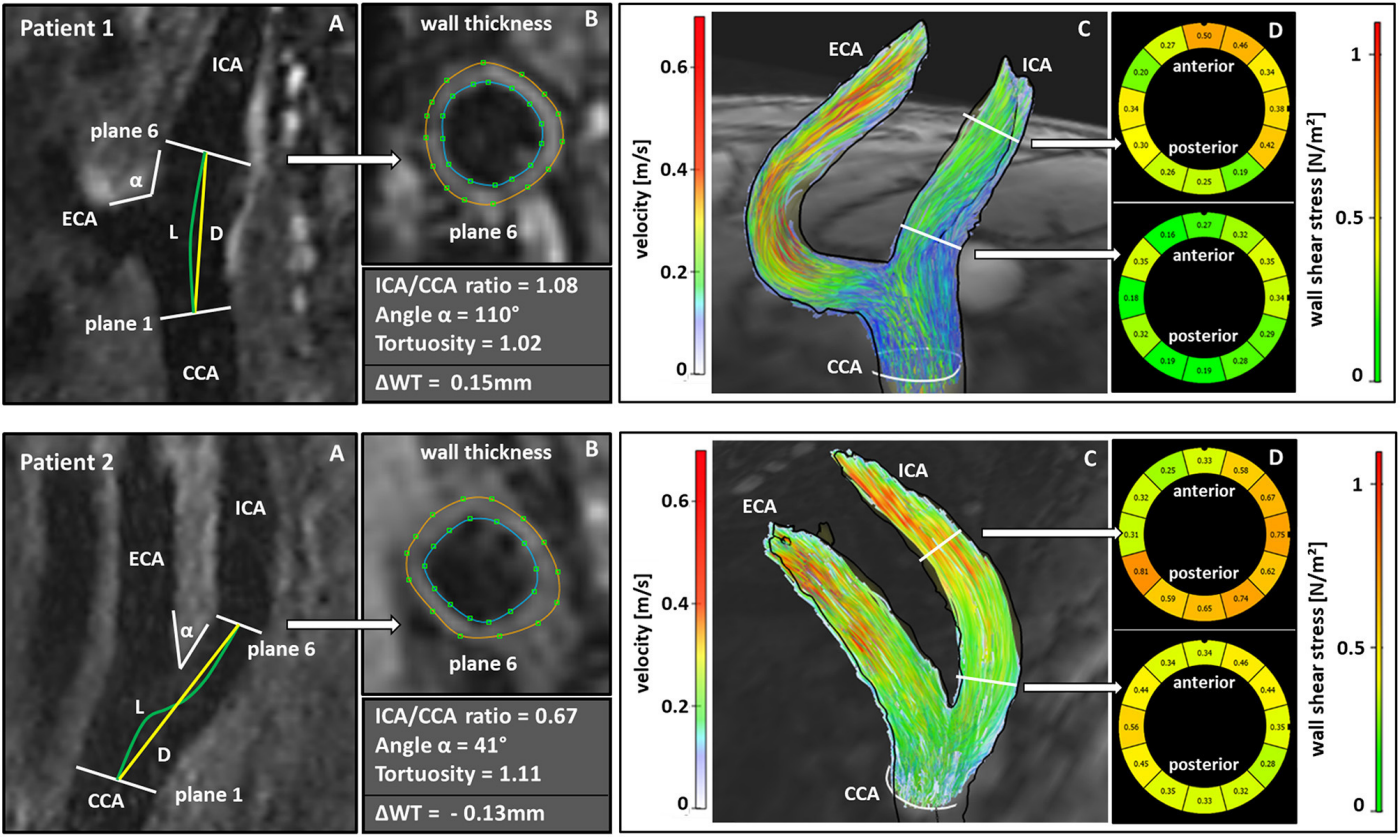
Comparison of geometric and hemodynamic properties associated with wall thickness progression (Patient 1) or plaque regression (Patient 2) during follow-up. Calculation of the ICA/CCA-ratio from the maximum diameters in plane 6 and plane1; L and D indicate the CCA-ICA distances along the lumen center (L) and the direct connection (D) for calculation of carotid tortuosity, α indicates bifurcation angle (A). Manual segmentation of wall thickness in the cross section corresponding to the white arrow (B). Streamlines represent absolute blood flow velocities in m/s derived from 4D flow MRI. (C). Wall shear stress analysis in the cross section corresponding to the white arrow (D). (Patient 1) showed an increase in wall thickness of 0.15 mm while (Patient 2) showed a decrease of wall thickness of 0.13 mm during follow-up. (Patient 1) had a higher ICA/CCA-ratio, a larger bifurcation angle, a lower tortuosity and showed lower wall shear stress values in the carotid bulb compared to (Patient 2) and compared to the values in the distal ICA. CCA, common carotid artery; ICA, internal carotid artery; ECA, external carotid artery; ΔWT, difference in wall thickness between follow-up and baseline examination.
